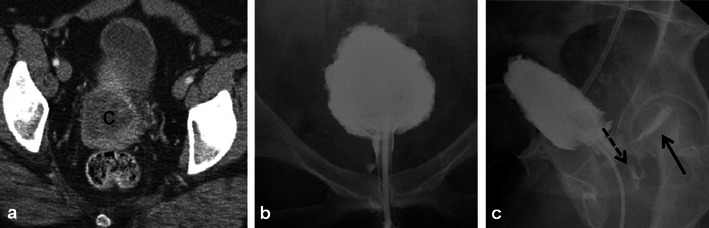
A 49-year-old woman post RT for locally advanced cervical squamous cell carcinoma depicted on pre-treatment axial CECT (a) which demonstrates the large necrotic cervical tumour (*C*). Following RT, a conventional cystogram was performed to evaluate for suspected fistula. Late filling AP (b) and oblique (c) radiographs of the urinary bladder demonstrate classic findings of radiation cystitis with a reduced capacity bladder that is elevated from the pelvic floor and has a rounded appearance. Note bladder wall thickening and trabeculation. Oblique radiograph (c) also depicts filling of the vagina (*dashed black arrow*) and rectum (*solid black arrow*) in keeping with complex radiation-induced vesicovaginal and rectovaginal fistulas
